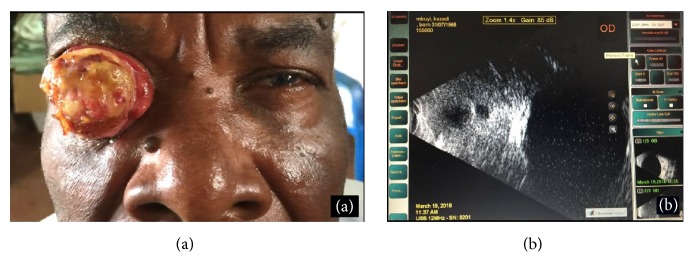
(a) Clinical aspect of the tumor. (b) Ultrasound examination.
